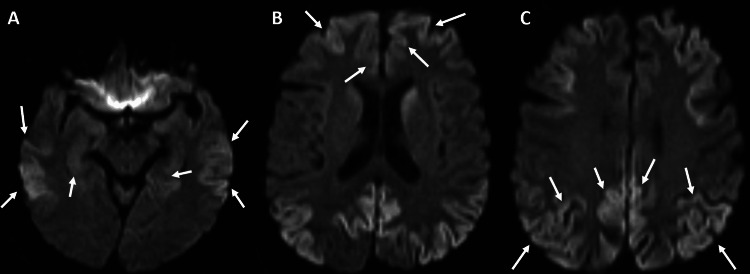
MRI (axial view) of Case 2 with cortical hyperintensity in DWI sequence The arrows point to the cortical ribboning of the bilateral (A) temporal, (B) frontal, and (C) parietal lobes. DWI: diffusion-weighted imaging
